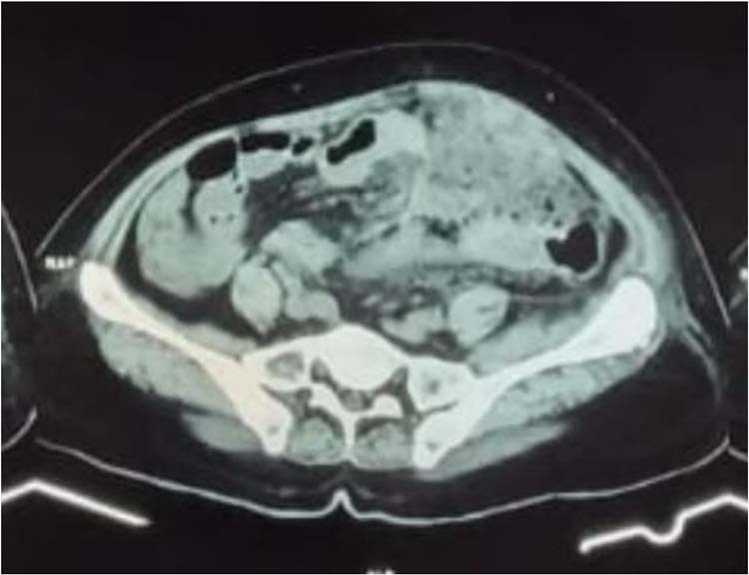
Abdominal CT scan with contrast showed transverse, descending to sigmoid colon wall thickening and significant contrast enhancement supporting colitis with mesenteritis.
